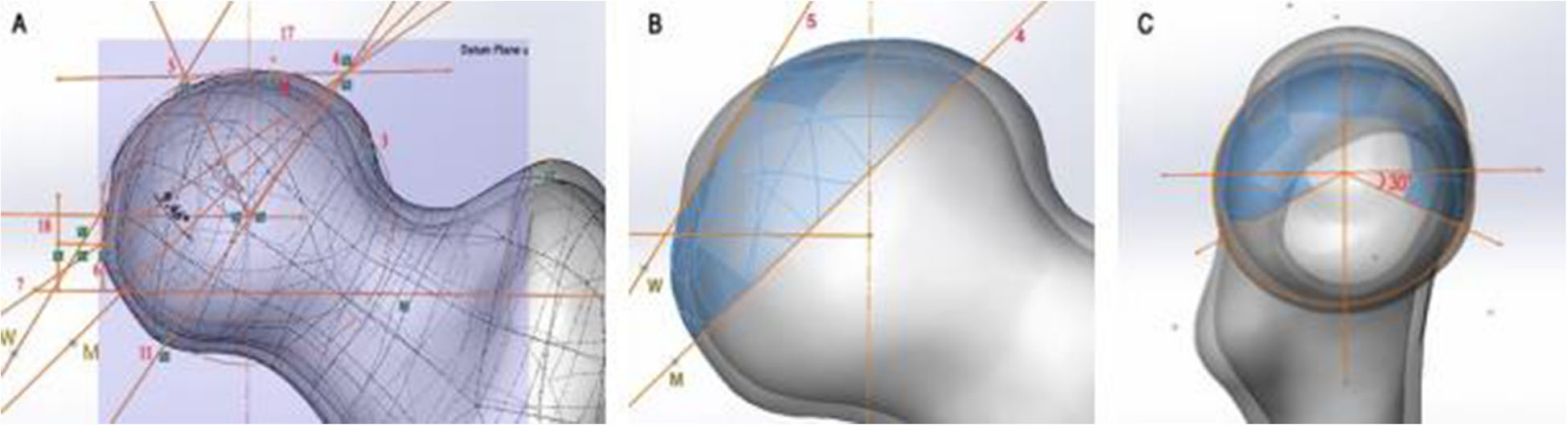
Calculation of the 3D weight-bearing area. a Transformation of the landmarks from the X-ray image to the datum plane α. b, c Definition of the limit line to trim out the shape of the weight-bearing surface
